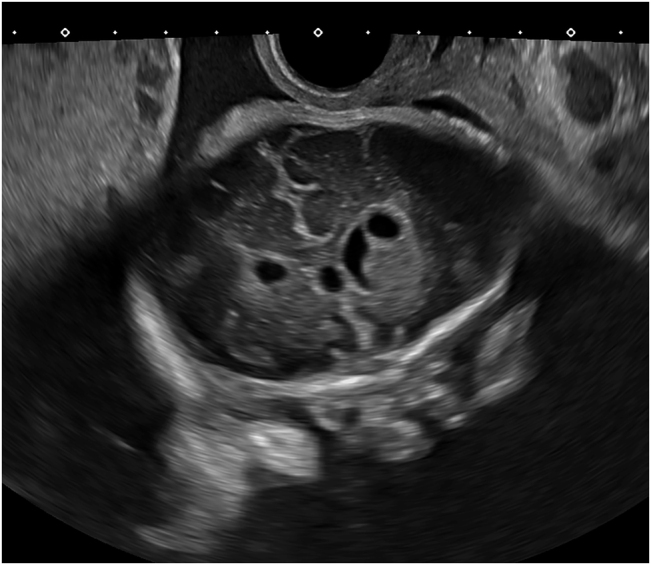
Transvaginal ultrasound at week 32 showing asymmetric ventriculomegaly and periventricular cyst.
